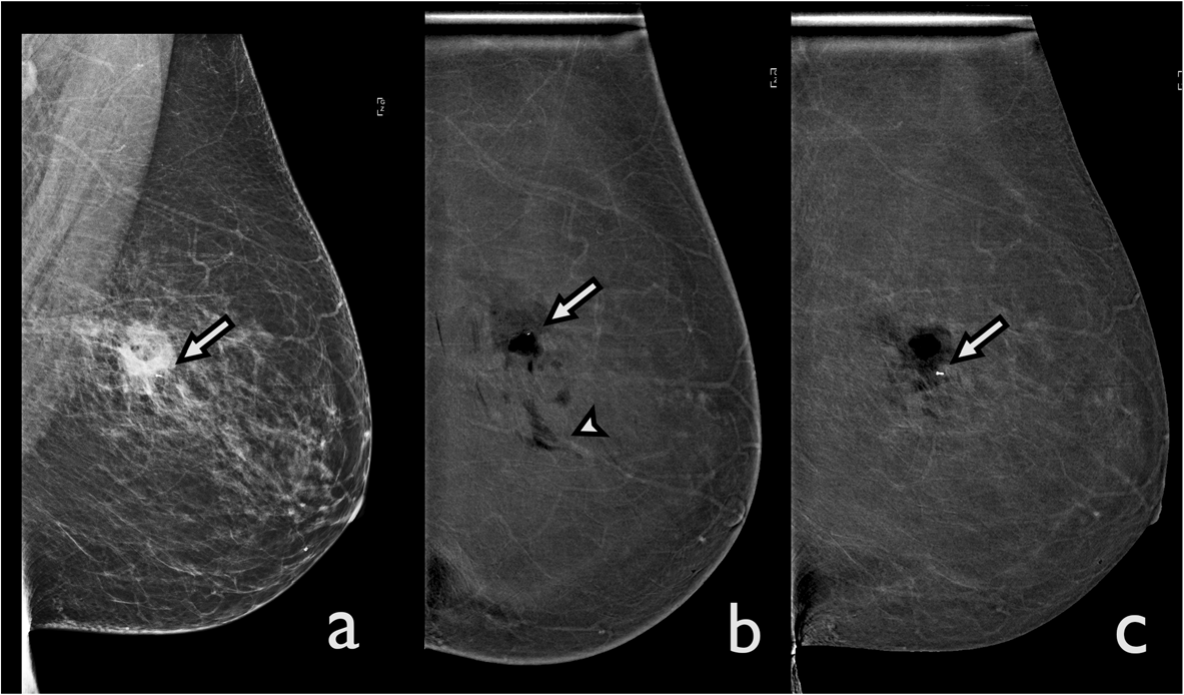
Motion artefact. A ductal carcinoma in situ was diagnosed in the left breast after an ultrasound-guided core needle biopsy. CEDM was performed for preoperative staging. a The LE image shows a post-biopsy hematoma with a marker (arrow). b The recombined CEDM image demonstrates that the margins of the hematoma are blurred secondary to motion artefacts. Some black bands are visible (arrowhead), and the post-biopsy marker appears to be in a different position from the previous image (arrow). c A new recombined CEDM image was performed. Here, the motion artefacts are no longer visible, and the marker appears to be in the right position (arrow)
